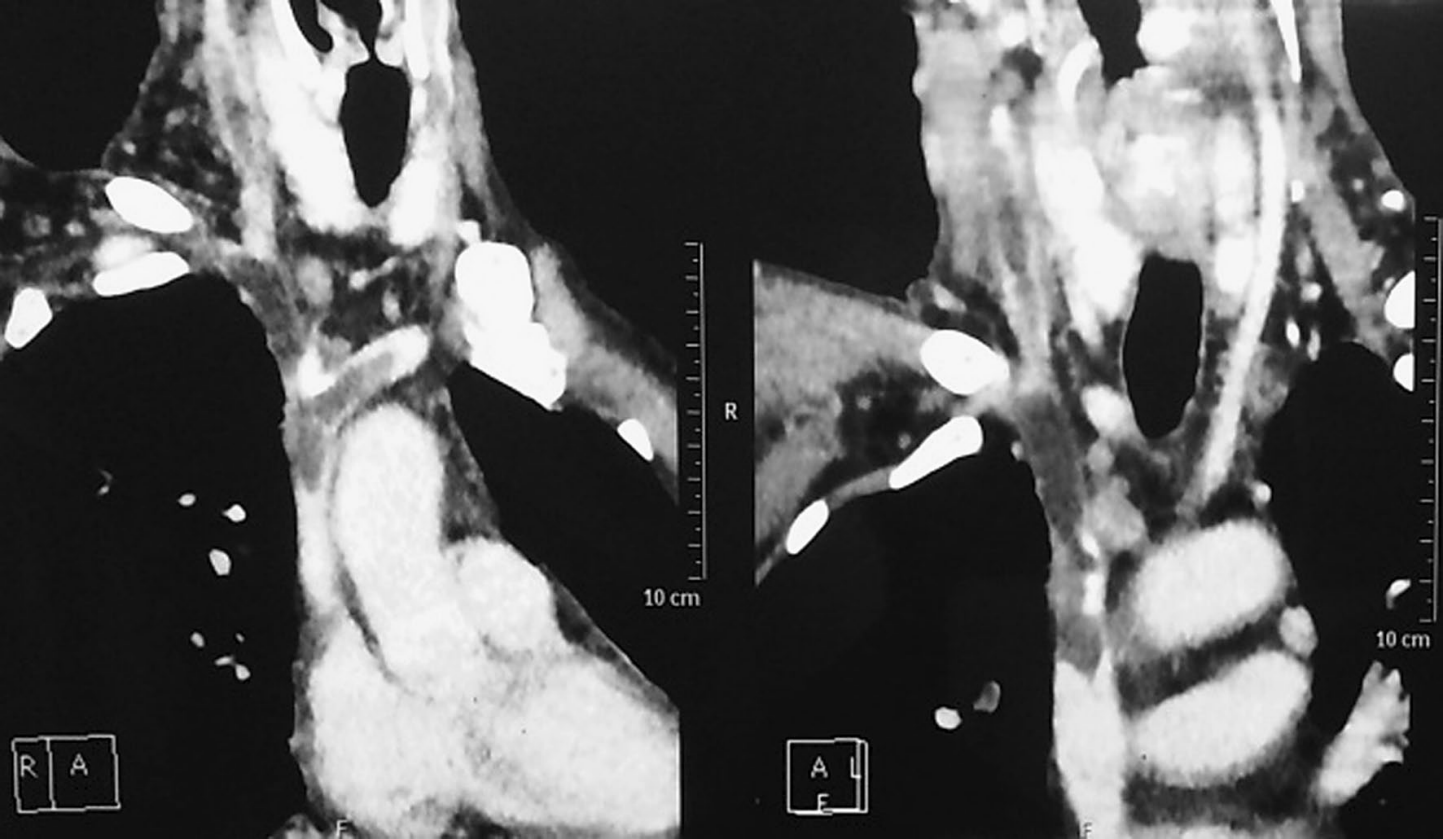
CT venography chest showing evidence of pulmonary thromboembolism and hypodense filling defect in the bilateral brachiocephalic veins *right* internal jugular vein
